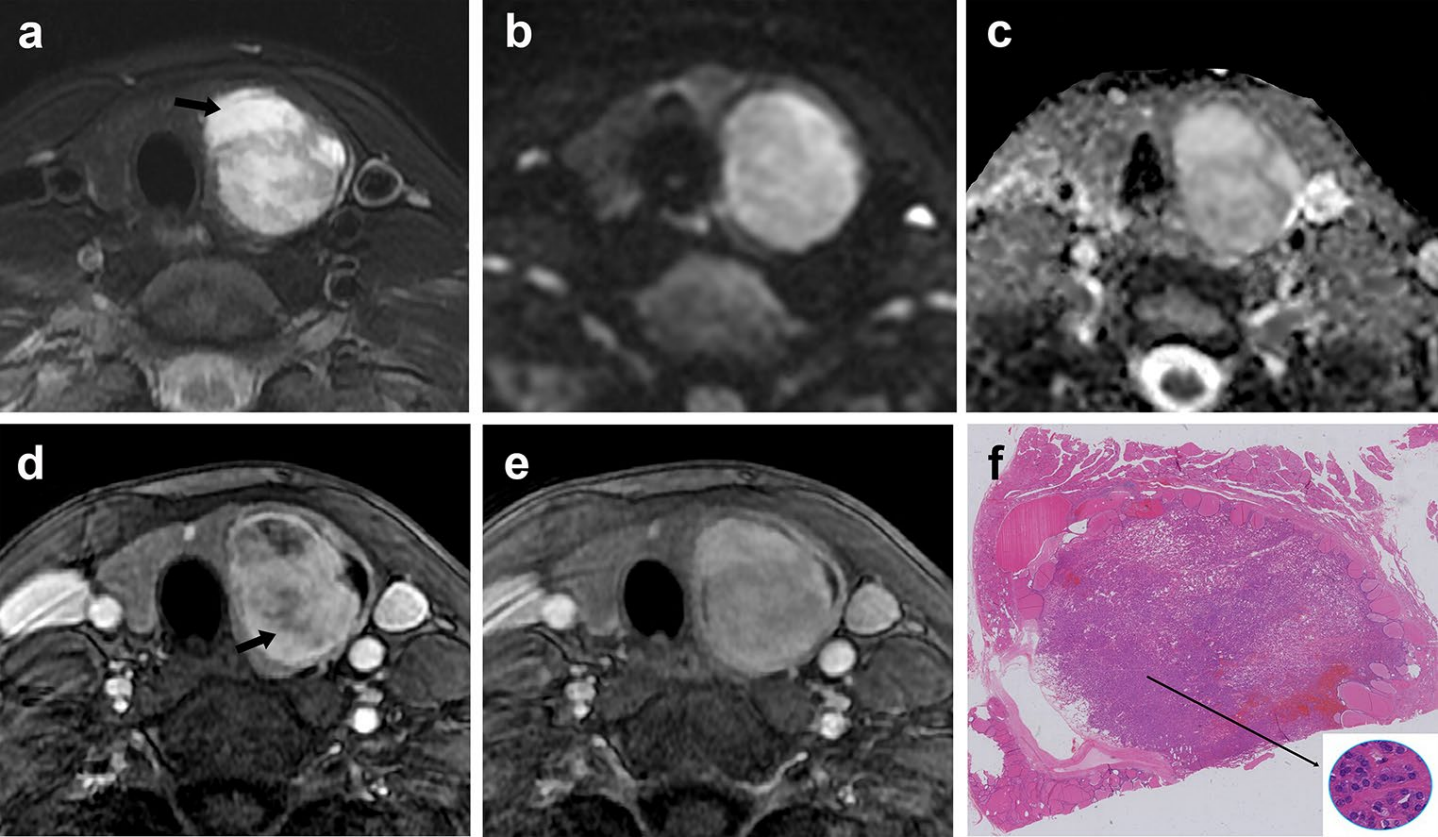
A 39-year-old woman presented with a thyroid follicular adenoma larger than 4 cm in the left lobe. Axial T2-weighted image a shows a heterogeneous mass and the present of hyperintense on T2WI with enhancement (black arrow). Axial DWI b and ADC c images show a hyperintense mass without restricted diffusion. Axial contrast-enhanced MRI (early phase) d shows a hyperintense enhancing mass and irregular fissure that did not enhance (black arrow). Axial contrast-enhanced MRI (delay phase) e shows a relatively homogeneous enhanced lesion with pseudocapsule, and fissure that do not enhanced in the early phase enhanced gradually. Pathological section analysis (HE, ×1) f shows densely distributed small follicles and relatively loose follicular cells (long black arrow, HE, ×40)
